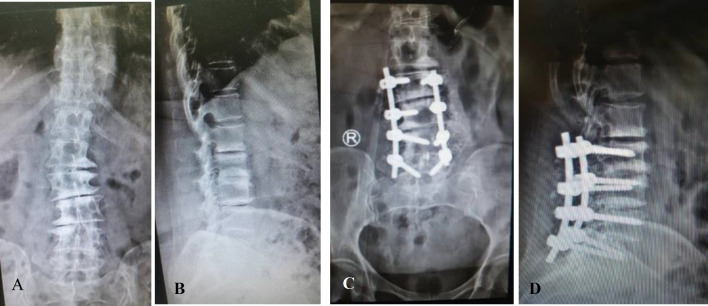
AP (A) and lateral (B) radiography of spine before surgery ,and AP (C)and lateral (D) radiography of spine after surgery
